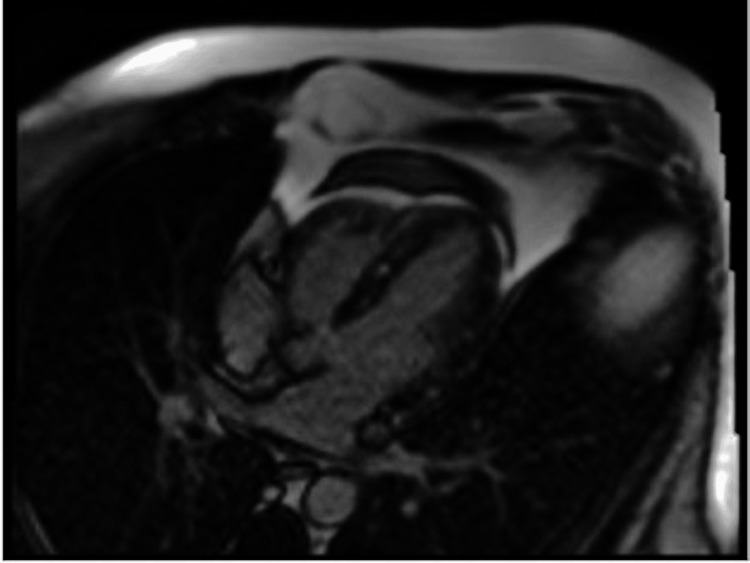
Follow-up MRI 4-chamber view showing multifocal, patchy, mid-myocardial late gadolinium enhancement
